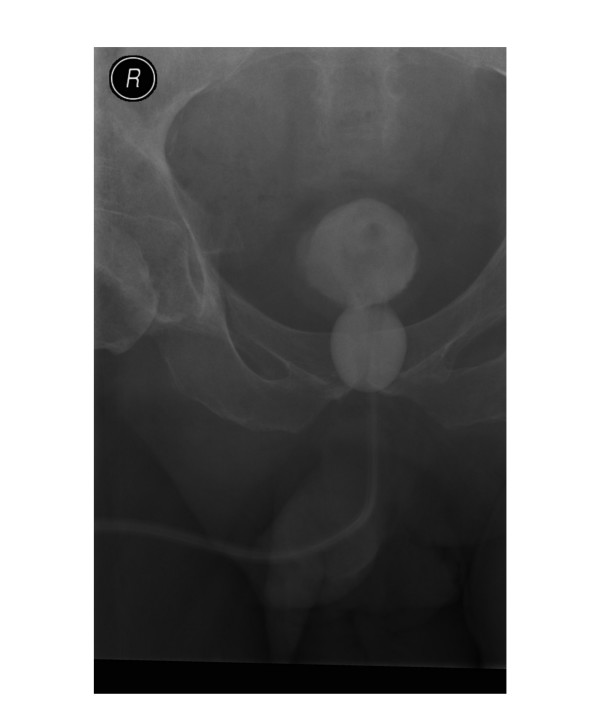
X-ray of pelvis, taken after injecting two ml of Ioversol (OPTIRAY 300) through balloon channel of Foley catheter and 50 ml of diluted contrast through the main lumen of Foley catheter in order to visualise Foley balloon and urinary bladder respectively: The outline of urinary bladder is seen clearly. A smaller circular opaque shadow, situated below the urinary bladder, represents the. Foley balloon. The Foley balloon is lying in posterior urethra. When a Foley catheter is positioned correctly, the Foley balloon should lie within the cavity of urinary bladder.
